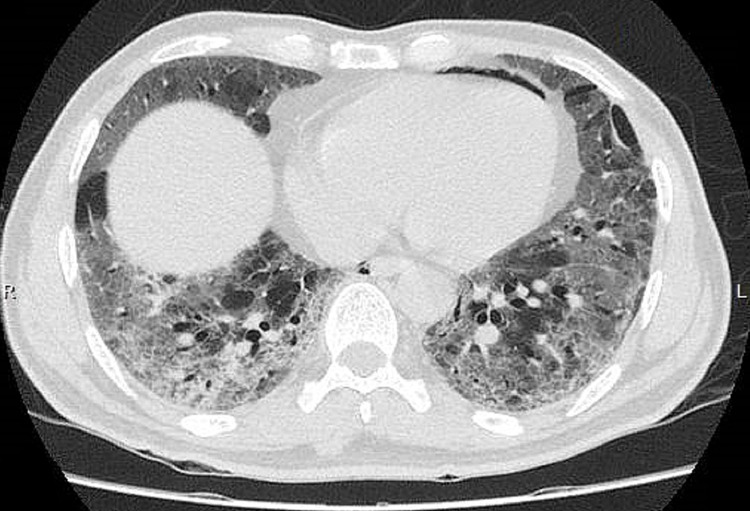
HRCT of the chest showing ground glass opacities involving both upper and lower lobes HRCT: High-resolution computed tomography
